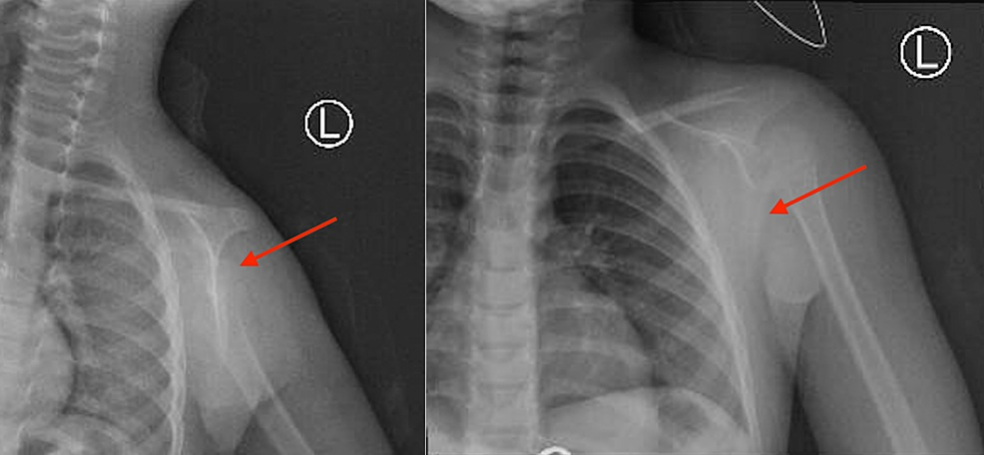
X-ray taken upon follow-up post-excision
